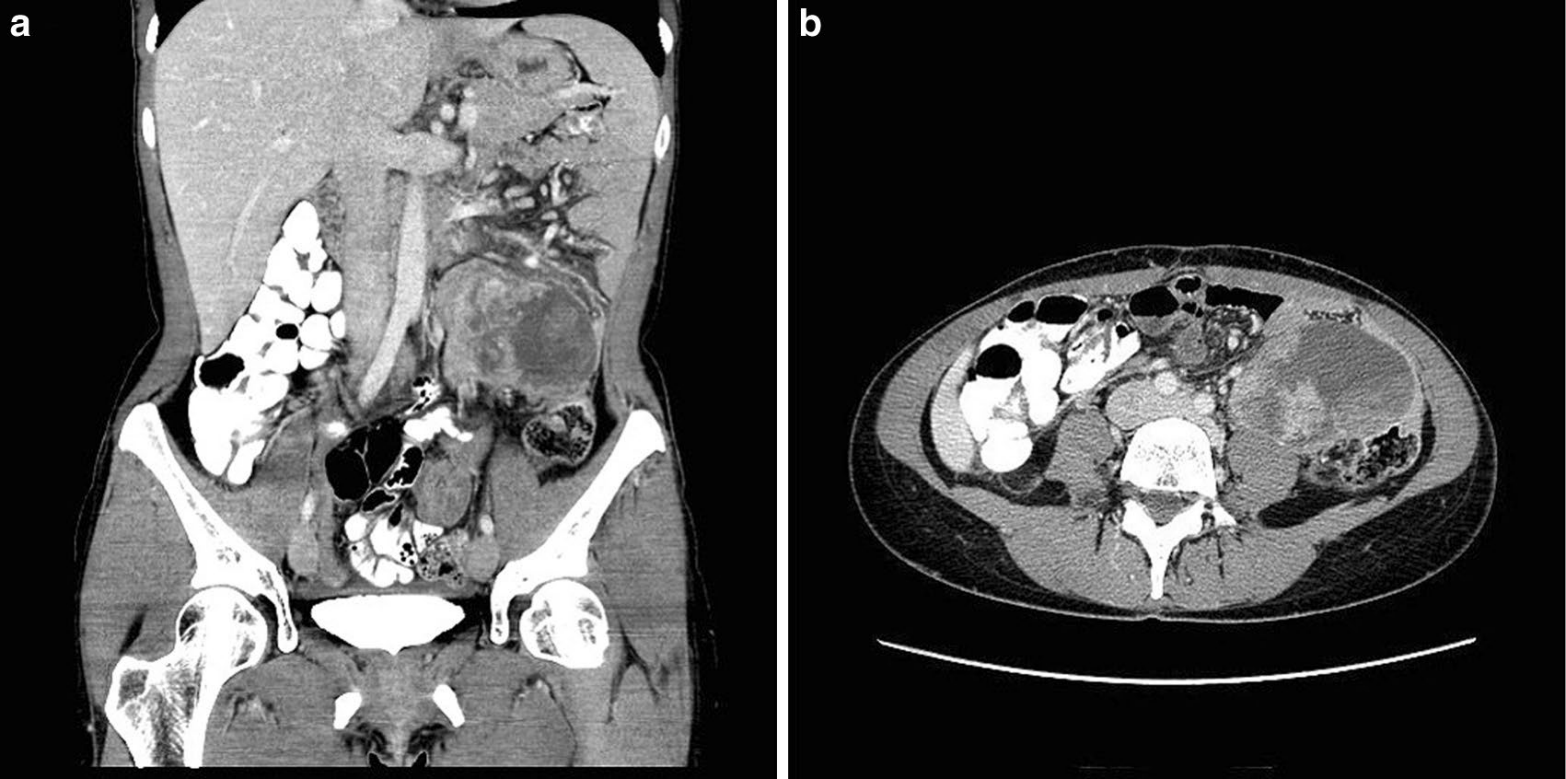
CT of the primary LMS. CT of the abdomen and pelvis showing an intraperitoneal tumor in the left quadrant measuring 8 × 7 × 7 cm (a coronal section). The tumor is located in the mesentery and is in close relation to both the small bowel and the sigmoid colon (b axial section). The low-density region within the tumor indicates necrosis
